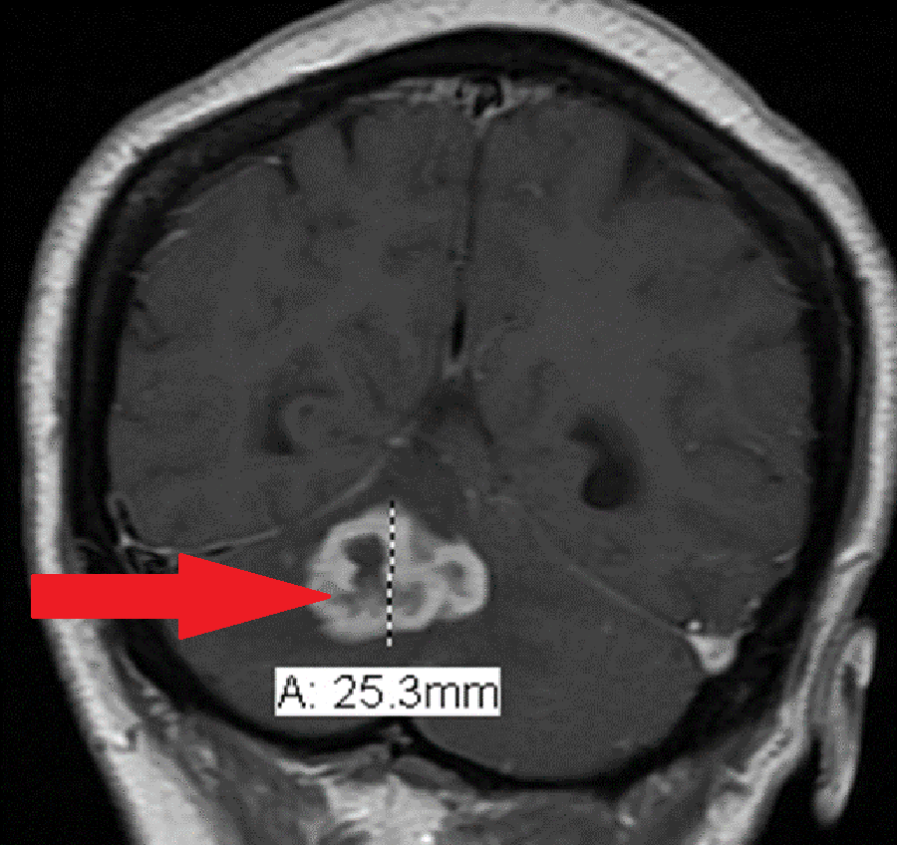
MRI of the brain (coronal view) shows a right cerebellar ring-enhancing lesion. MRI of the brain from a vertical, frontal plan (coronal view) showing a large (25.3 mm diameter) ring-enhancing lesion in the patient's cerebellum, which is indicated by the arrow. MRI - magnetic resonance imaging.
